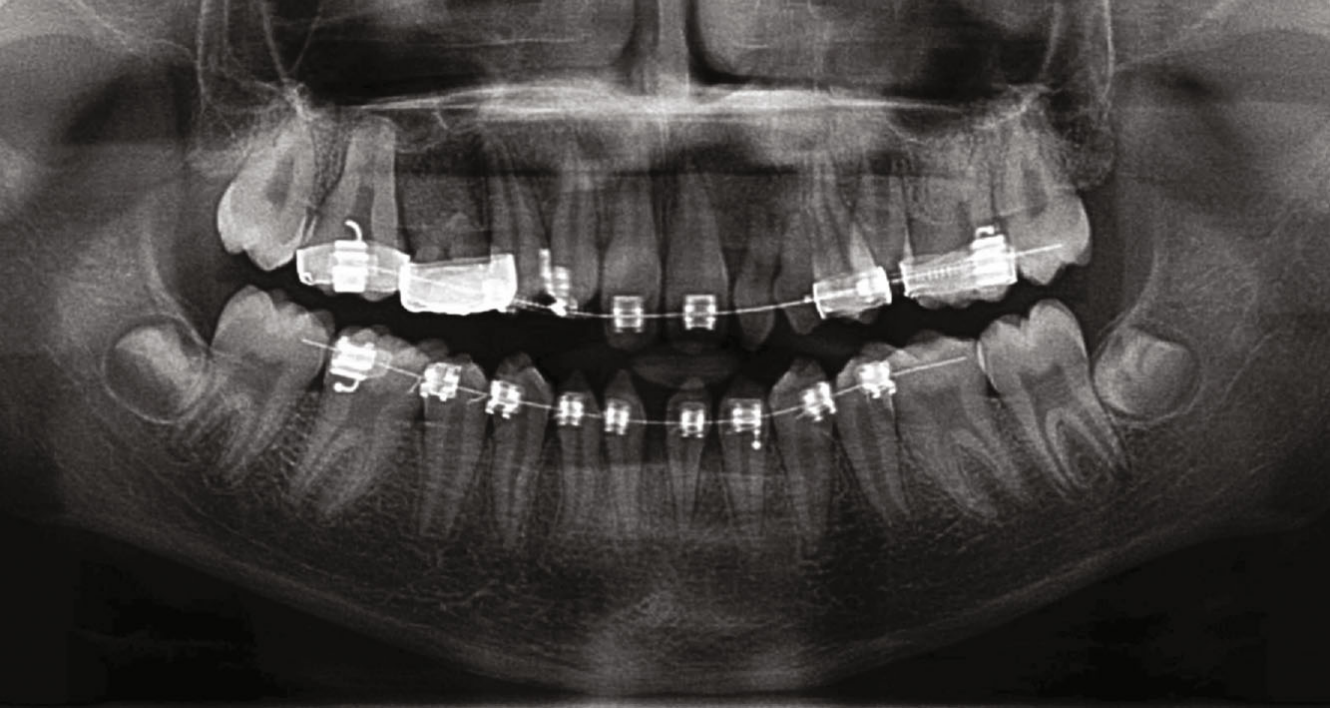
The “near-end radiograph” (digital dental panoramic tomograph).
